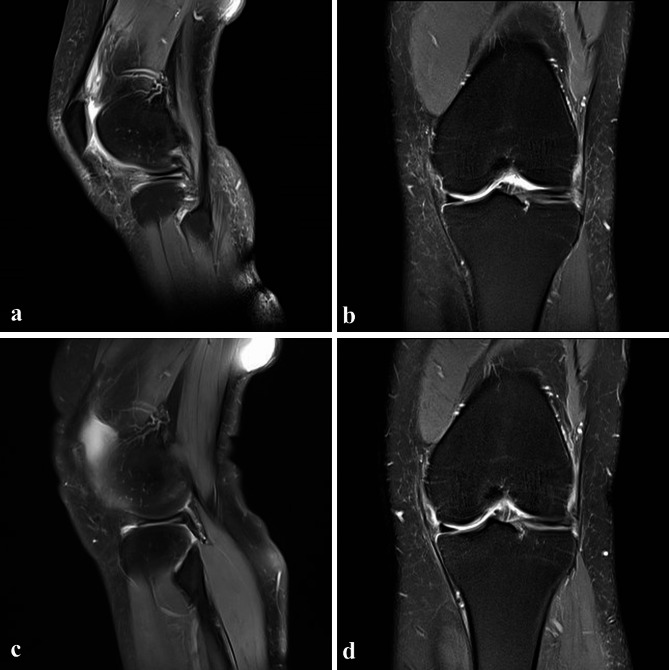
(a and b) Horizontal tear of the involved LMAH is observed in a sagittal and coronal MRI in a 26-year-old female patient. (c and d) Sagittal and coronal MRIs after 3 months of surgery reveal the resected inferior leaf of the LMAH. LMAH, lateral meniscus anterior horn; MRI: magnetic resonance imaging
